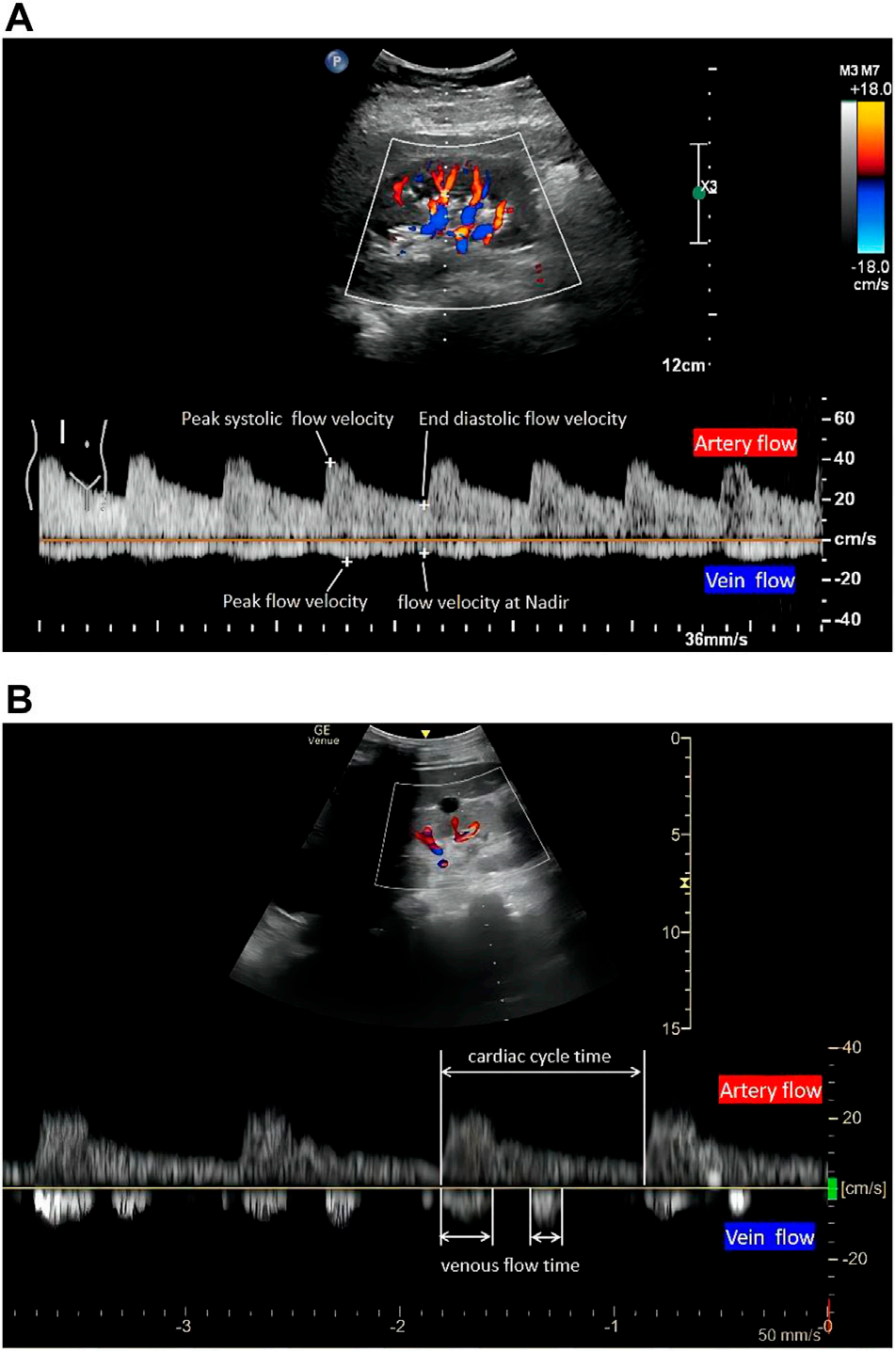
The quantitative evaluation of renal hemodynamics by pulse wave Doppler in the interlobar vessels of the right kidney. Intrarenal artery flow (upward Doppler signals) and vein flow (downward Doppler signals). A continuous venous flow pattern in (A) and a biphasic pattern in (B) (1). RRI, (peak systolic flow velocity-end diastolic flow velocity)/peak systolic flow velocity. (2) PI, (peak systolic flow velocity-end diastolic flow velocity)/mean flow velocity. (3) VII, (peak flow velocity-flow velocity at nadir)/peak flow velocity. (4) RVSI, (cardiac cycle time-venous flow time)/cardiac cycle time. RRI, renal resistive index; PI, renal pulsatility index; VII, venous impedance index; RVSI, renal venous stasis index.
